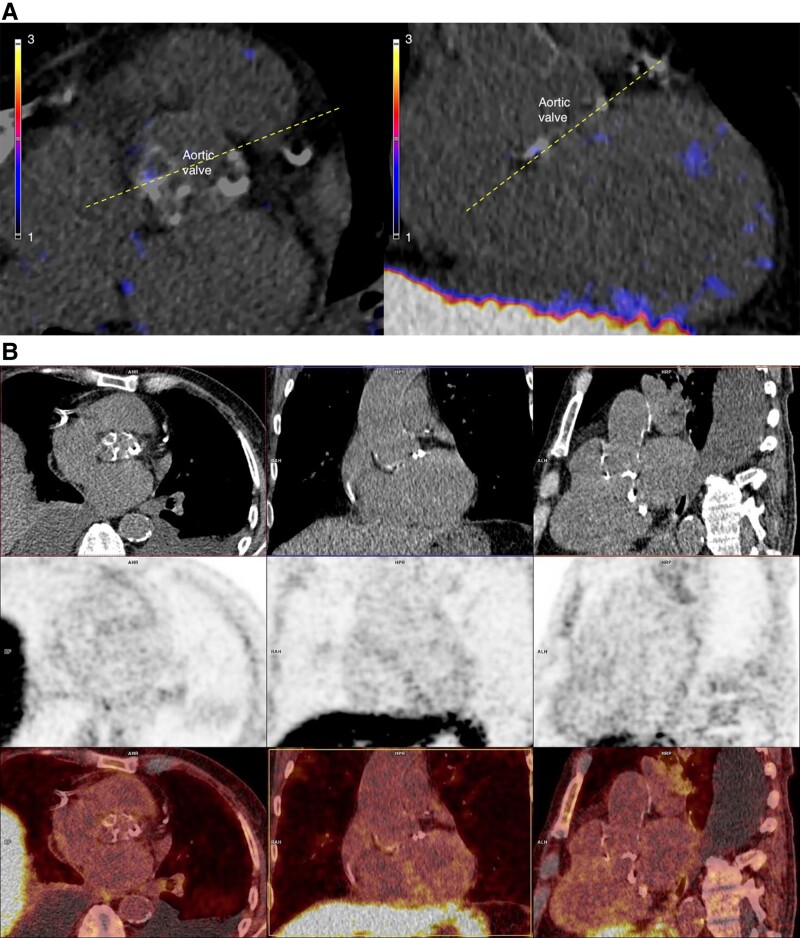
(*A*) [^64^Cu]Cu-DOTATATE positron emission tomography/computed tomography of Patient 2. Perpendicular positron emission tomography/computed tomography fusion images of the aortic valve without an increased uptake of [^64^Cu]Cu-DOTATATE. The myocardium in the ventricle has very low tracer uptake. The dotted lines indicate two image planes. LV, left ventricle; RV, right ventricle. (*B*) Positron emission tomography/computed tomography images from Patient 2 showing an uptake of [^64^Cu]Cu-DOTATATE in cross-sectional, coronal, and sagittal views, respectively. Top row: computed tomography images; middle row: positron emission tomography images; bottom row: fused positron emission tomography/computed tomography images.
